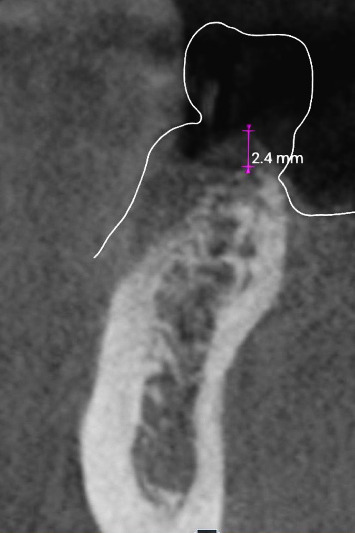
Regio #30-cross-sectional image from CBCT with visualization of the soft tissue height before implantation.
